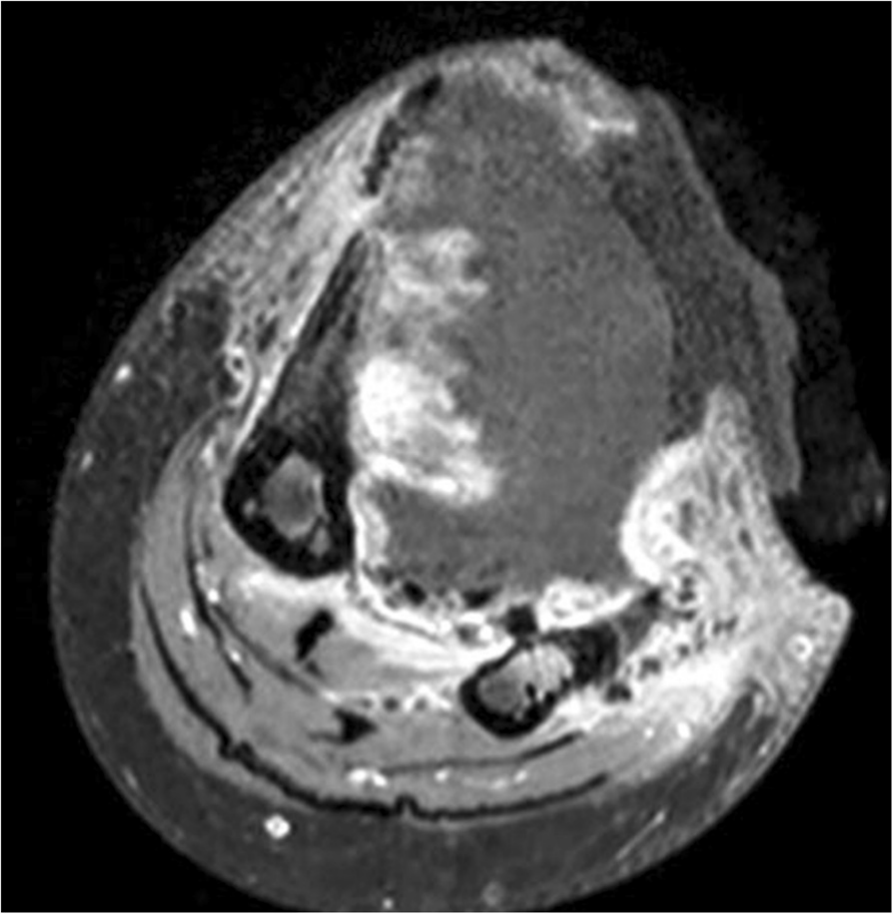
Five-year follow-up magnetic resonance imaging postcontrast-enhanced axial T1-weighted fat-suppressed demonstrated ruptured skin.
